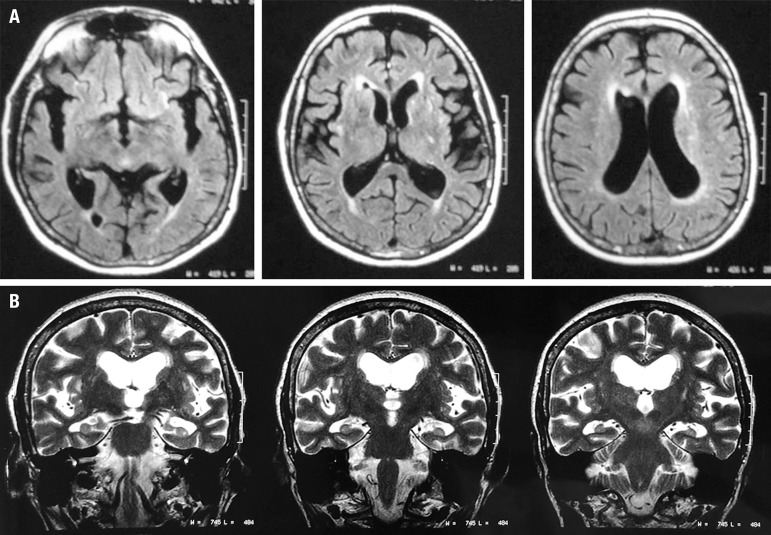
[A] Magnetic Resonance Imaging (MRI)* [B]
